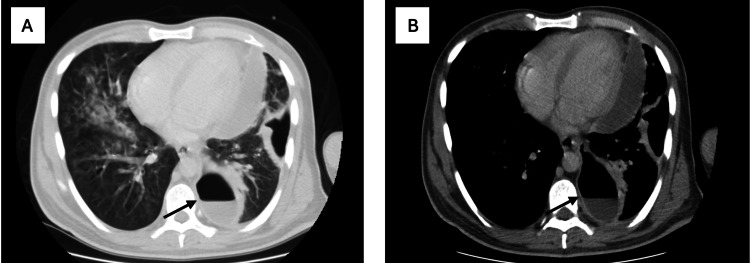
(A) Chest CT scan (lung window) showing bilateral parenchymal infiltrations suggestive of pneumonia. (B) Chest CT scan (mediastinal window) showing a left-sided cavitation communicating between the gastroesophageal junction (GEJ) and the left lung, suggestive of a gastropleural fistula (GPF).
